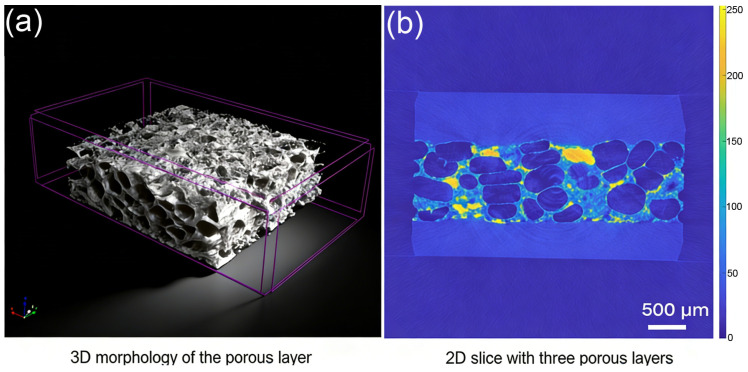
Laboratory X-ray CT imaging of PEEK implants. (a) 3D morphological reconstruction. (b) Representative 2D slice through the center.
